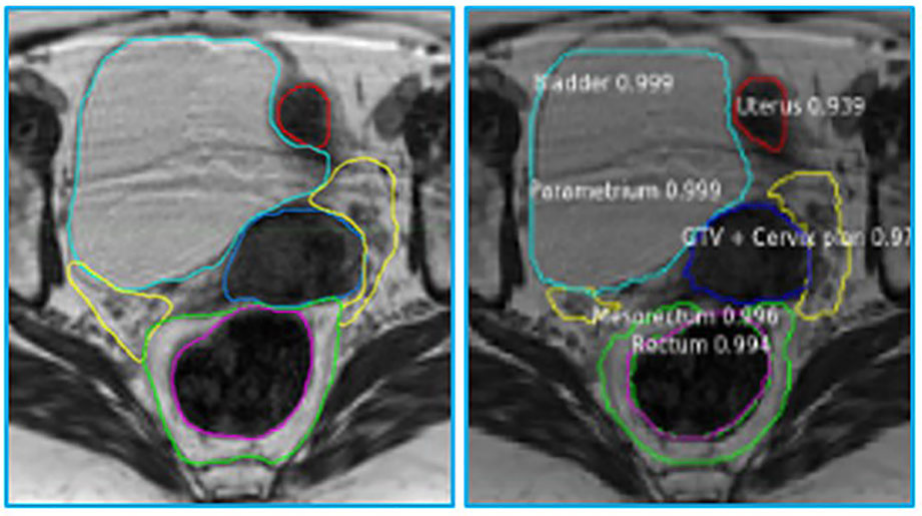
Manual (left) and automatic (right) contours on the original MRI image from a patient with cervical cancer. The contours generated by MASK R-CNN contain a confidence estimate, a number between 0 and 1, with 0 representing mimmum and 1 maximum confidence that the class, assigned to the segmented volume is accurate. Please refer to Figure 1 legend for color scheme.
